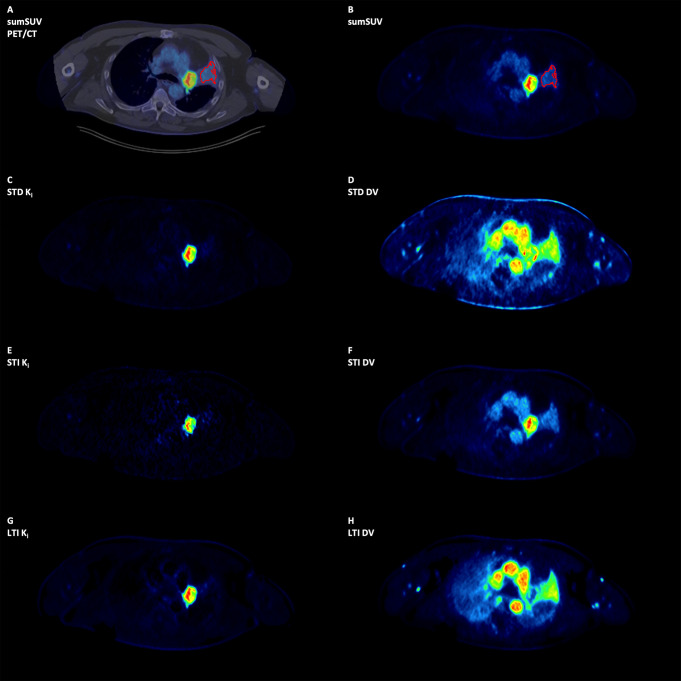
Patient with a primary lung tumor and atelectasis. The Patlak images are true negative compared to the sumSUV image. In the sumSUV (A fused PET-CT and B) images there is [^18^F]FDG enhancement in the atelectasis, but not in any of the Patlak K_i_ images (C, E, G). All DV images (D, F, H) showed enhanced contrast in the atelectasis and variable contrast in the primary tumor depending on the method used. In particular, LTI DV images (H) showed a rim-like uptake in the primary lung tumor. The DV enhancement in the atelectasis is related to higher perfusion-related [^18^F]FDG transport but not phosphorylation, explaining the enhanced SUV
